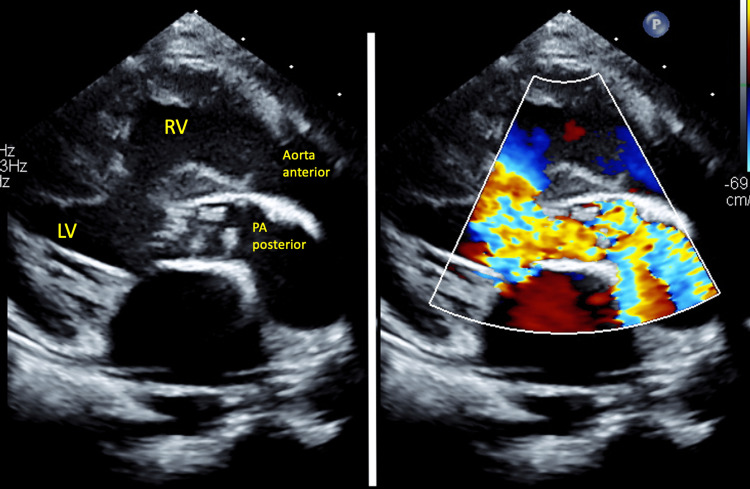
Preoperative echocardiogram (parasternal long axis view) showing the relation of the aorta to the pulmonary artery LV, left ventricle; PA, pulmonary artery; RV, right ventricle
